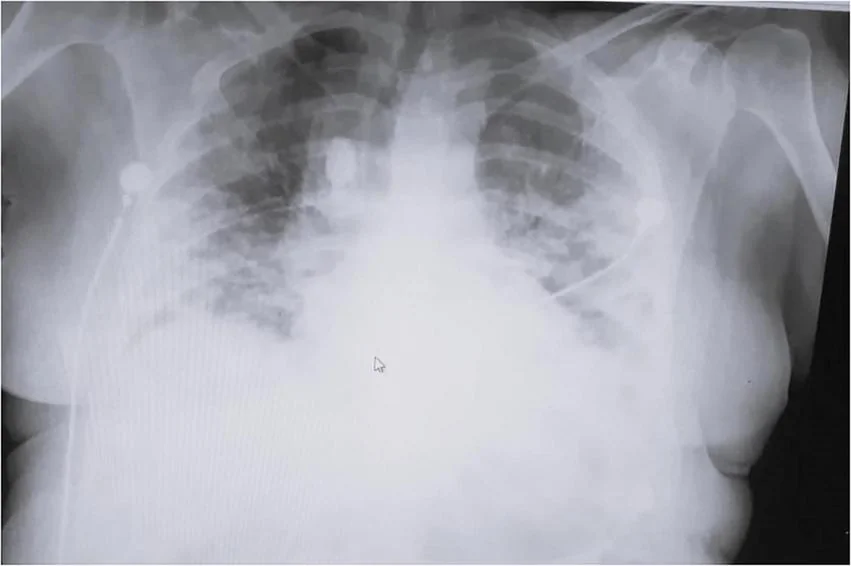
Portable anteroposterior chest radiograph on hospital admission. Portable anteroposterior (AP) chest radiograph obtained on hospital admission before initiation of invasive mechanical ventilation. The image demonstrates bilateral diffuse patchy air-space opacities predominantly involving the mid and lower lung fields, consistent with severe COVID-19 pneumonia. No pleural effusion or pneumothorax is evident on this radiograph. These imaging findings provided the initial radiological context for ICU admission and subsequent invasive mechanical ventilation.
